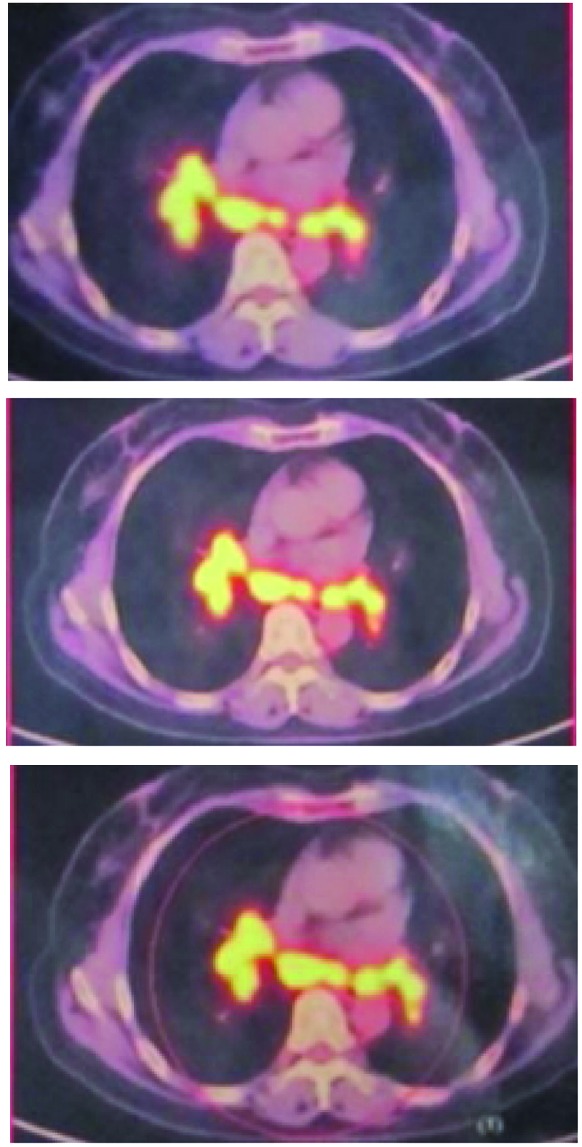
Positron emission tomography and computed tomography images of the mediastinal and hilar lymph nodes.
